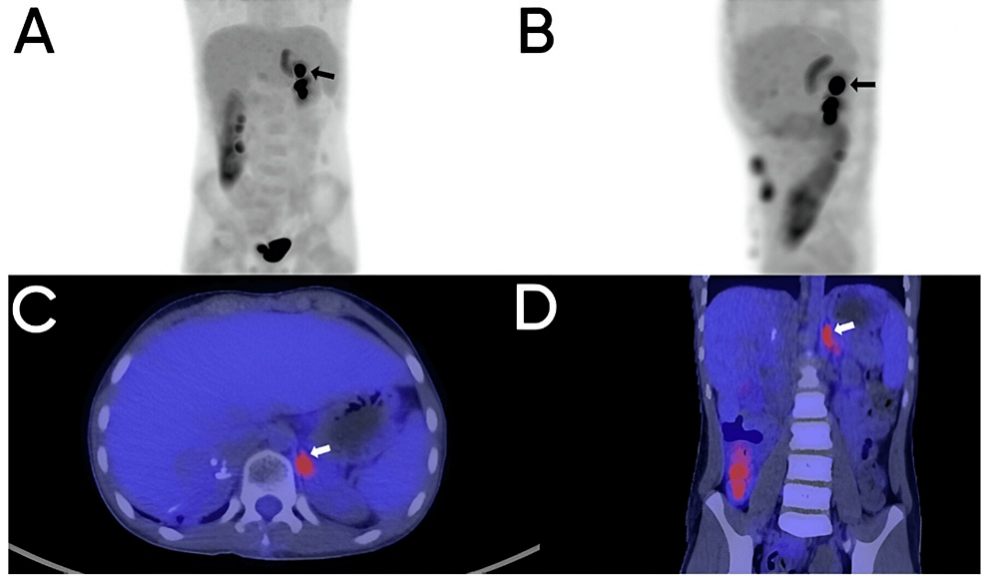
Positron emission tomography with fluorodeoxyglucose (PET-FDG). Maximum intensity projection (MIP) volumetric images of PET with FDG (A and B), fusion of plain computed tomography and PET-FDG in the axial plane (C) and coronal plane (D). Focal FDG uptake in the left adrenal gland is evident in the MIP volumetric images of PET with FDG (A and B). In the fusion of CT and PET-FDG, it is confirmed that the focus of increased uptake corresponds to the left adrenal gland with a maximum SUV of 3.95 (arrow in C and D).
